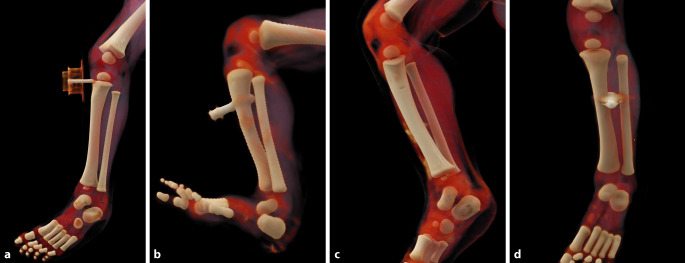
Typische Fehllagen in 3D-Rekonstruktionen aus CT-Daten verstorbener Kindern. a 9 Monate alter männlicher Säugling mit Fehllage der Kanüle in der rechten Epiphysenfuge der linken Tibia. b Am Tag der Geburt verstorbener weiblicher Säugling mit Perforation der Kanüle durch die dorsale Kortikalis. c 6 Monate alter männlicher Säugling mit lateraler Kortikaliskerbe durch i.o.-Kanüle in der linken Tibia. d 2 Monate alter männlicher Säugling mit Fehllage zwischen Tibia und Fibula links
